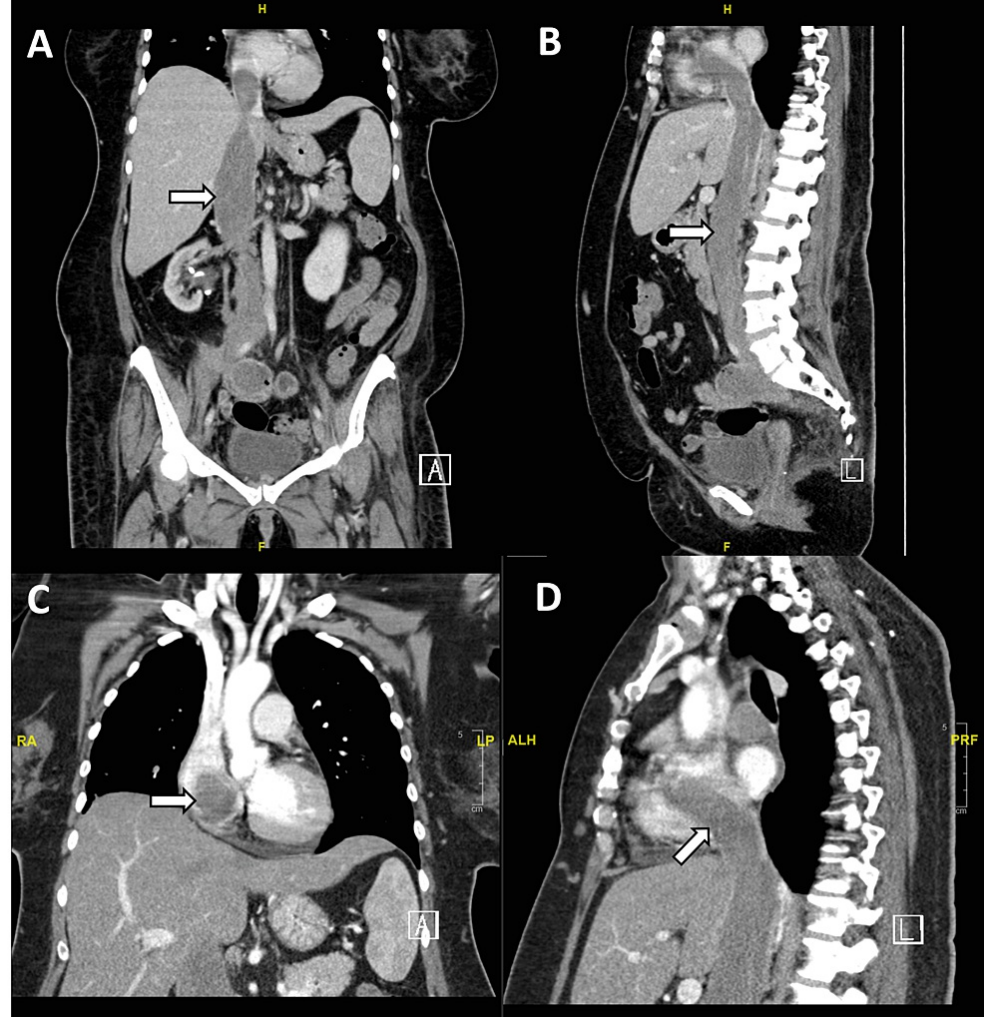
CT scan with contrast (coronal and sagittal views) of the chest, abdomen, and pelvis. CT scan views (A,B) demonstrate a soft tissue mass in the right psoas muscle with near total occlusion of the inferior vena cava by thrombus (white arrows in A,B), and (C,D) extension into the right atrium (white arrows in C,D).
